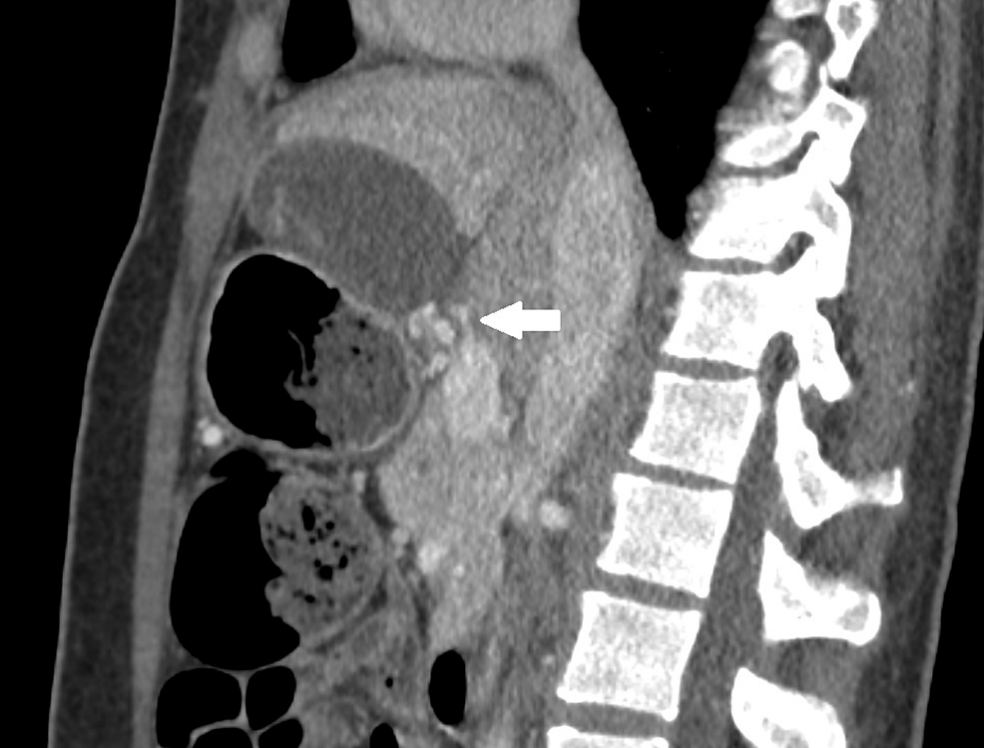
Sagittal CT scan in arterial phase shows twisted gallbladder vascular pedicle with swirl appearance (arrow)
